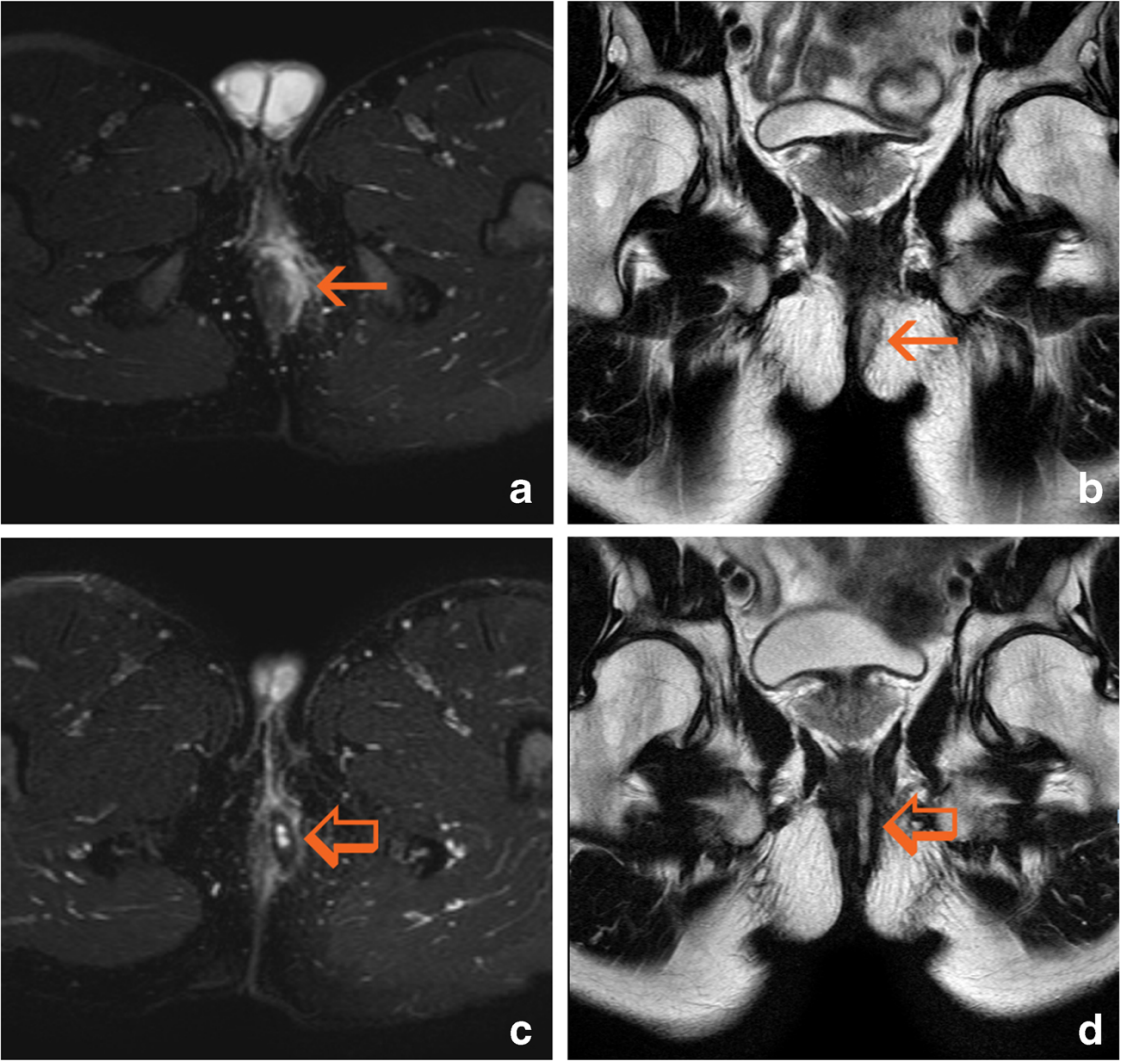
Anal ulcer. Diffuse hyperintensity of the left anal margin is present on T2-weighted images, and enhancement is present in the post-gadolinium sequence (arrows). A true fistula developed during follow-up (open arrows). a Oblique-transverse fat-supressed T2-weighted image. b Oblique-coronal T2-weighted image. c Oblique-transverse fat-supressed T2-weighted image. d Oblique-coronal T2-weighted image
